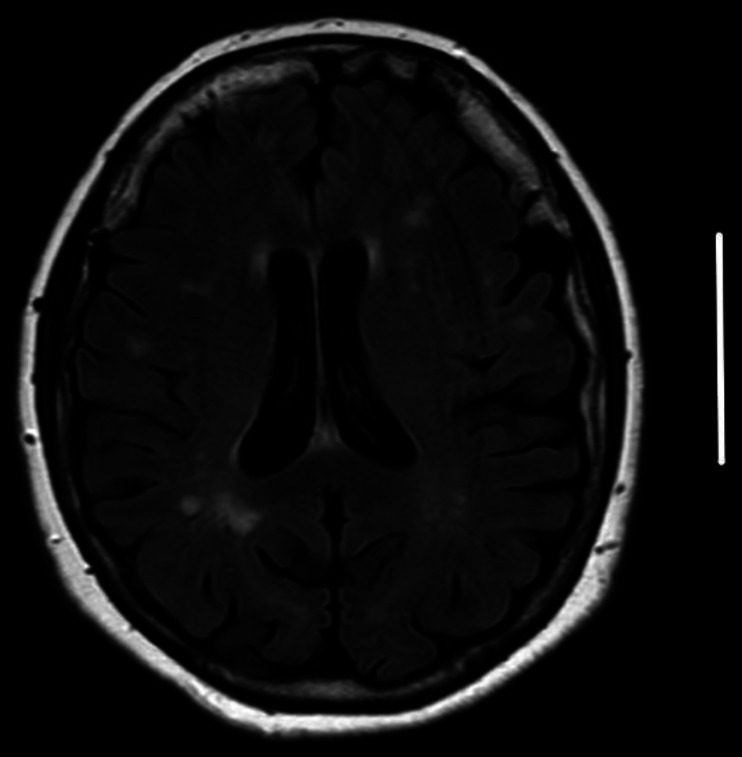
White matter hyperintensity on T2 fluid-attenuated inversion recovery magnetic resonance imaging sequence (scale bar, 5 cm; source: Medical Imaging Centre, Semmelweis University). Based on our white matter hyperintensities evaluation protocol, this patient was categorized as posterior cerebral artery region right dominant.
